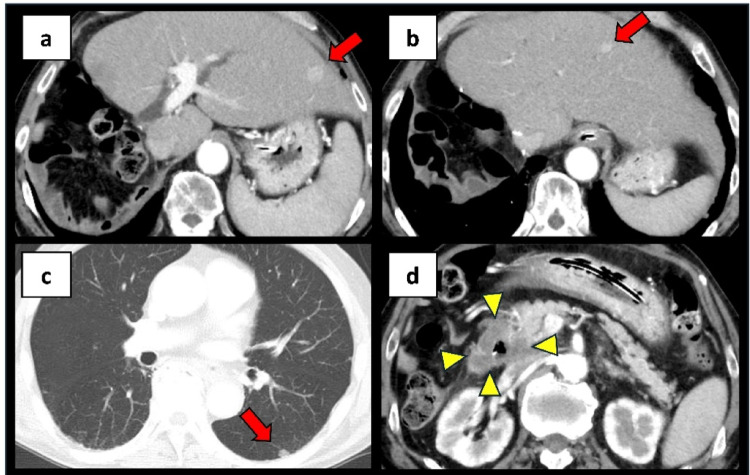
Contrast-enhanced computed tomography at presentation. Two nodular lesions are observed in the left hepatic lobe (arrows) and a solitary nodule is seen in the left lower lobe of the lung (arrow) (a–c). In addition, circumferential wall thickening of the descending duodenum is observed (arrowheads) (d)
